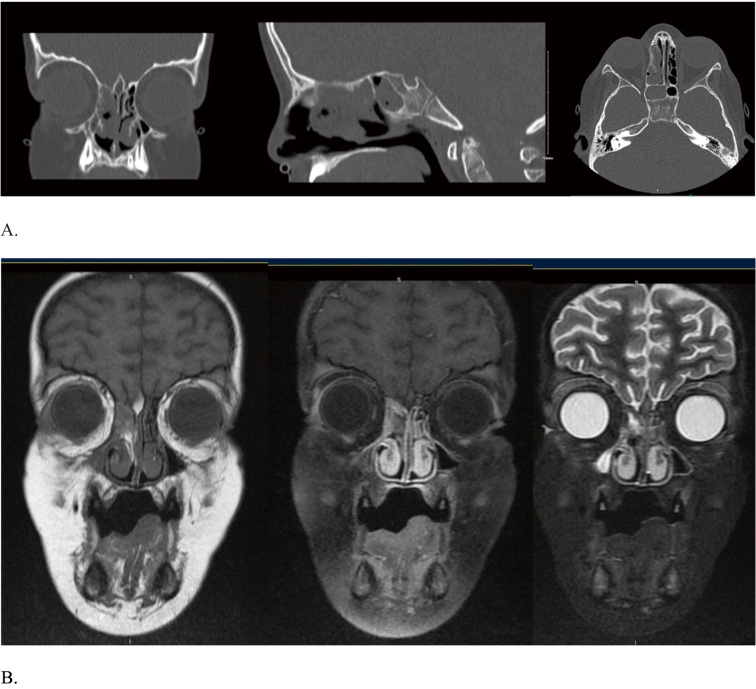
A) After the first surgery, regression of the previously visualized right anterior ethmoidal hyperdense opacification with residual peripheral hyperdense opacification was seen in different views. B) MRI with contrast prior to the second surgery showing low signal intensity on T1 (left), contrast and fat suppression on T1 (middle), and high signal intensity on T2 (right). The right anterior ethmoid sinuses showing residual mucosal thickening and sinus fluid, and bilateral opacification of the maxillary sinuses with low intensity in T1 (Left), minimal enhancement post contrast more in the right side and high intensity in T2 (right).
